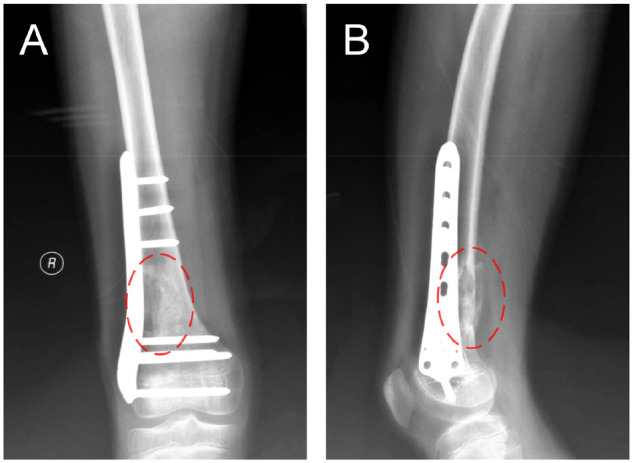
Frontal (A) and lateral (B) views of plain radiography at 1 month after the operation (the surgical site is noted by dashed circle in each picture).
